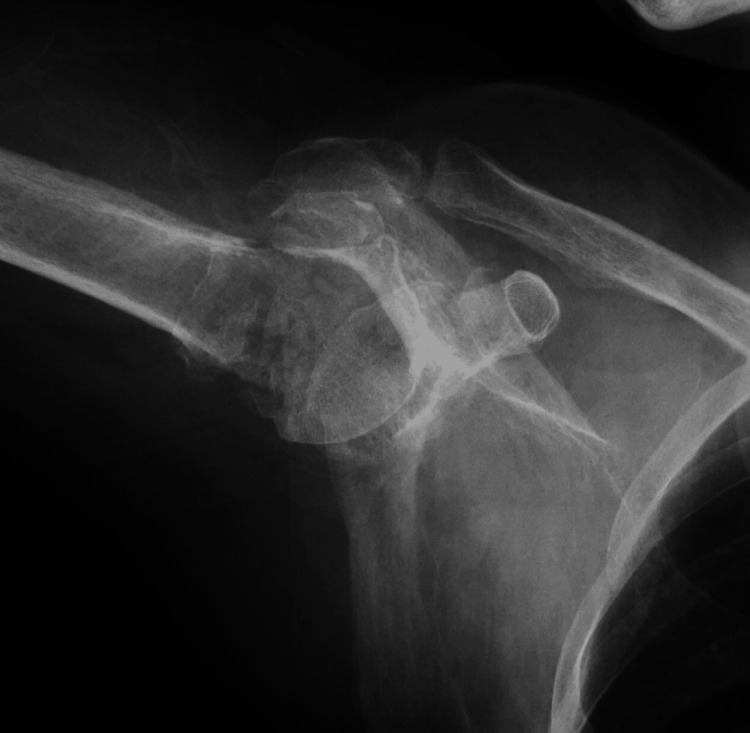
Plain radiograph on admission showing multiple fractures of the right upper extremity Right shoulder fracture-dislocation, proximal right humeral fracture, and right clavicle fracture are identified.
